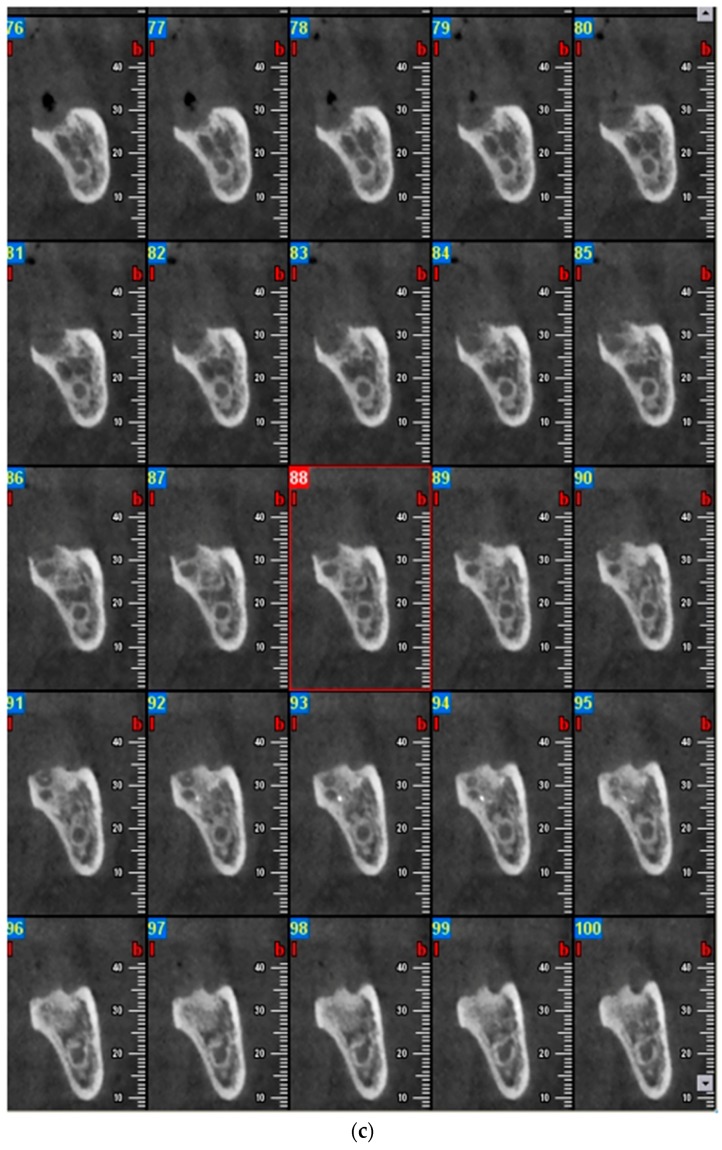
Case 1: Cone-beam computer tomography images: (a) 3D reconstruction; (b) Panorex; (c) CT scan images of the bone exposition.
